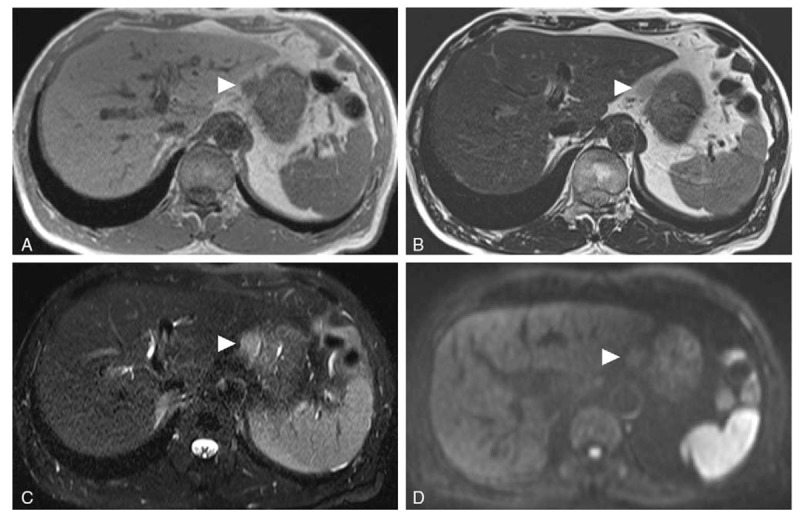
Representative MRI images of a capillary hemangioma located in the lesser omentum. A: T1WI in the axial plane showing a mass (arrowhead) by the lesser curvature with low SI equivalent to that in the muscles or spleen. B: T2WI showing relatively high SI of the mass (arrowhead). C: Fat-suppressed T2WI showing no decrease in the SI of the mass (arrowhead). D: Diffusion-weighted MRI showing isointensity of the mass (arrowhead) compared with the liver. MRI = magnetic resonance imaging, SI = signal intensity, T2WI = T2-weighted imaging.
